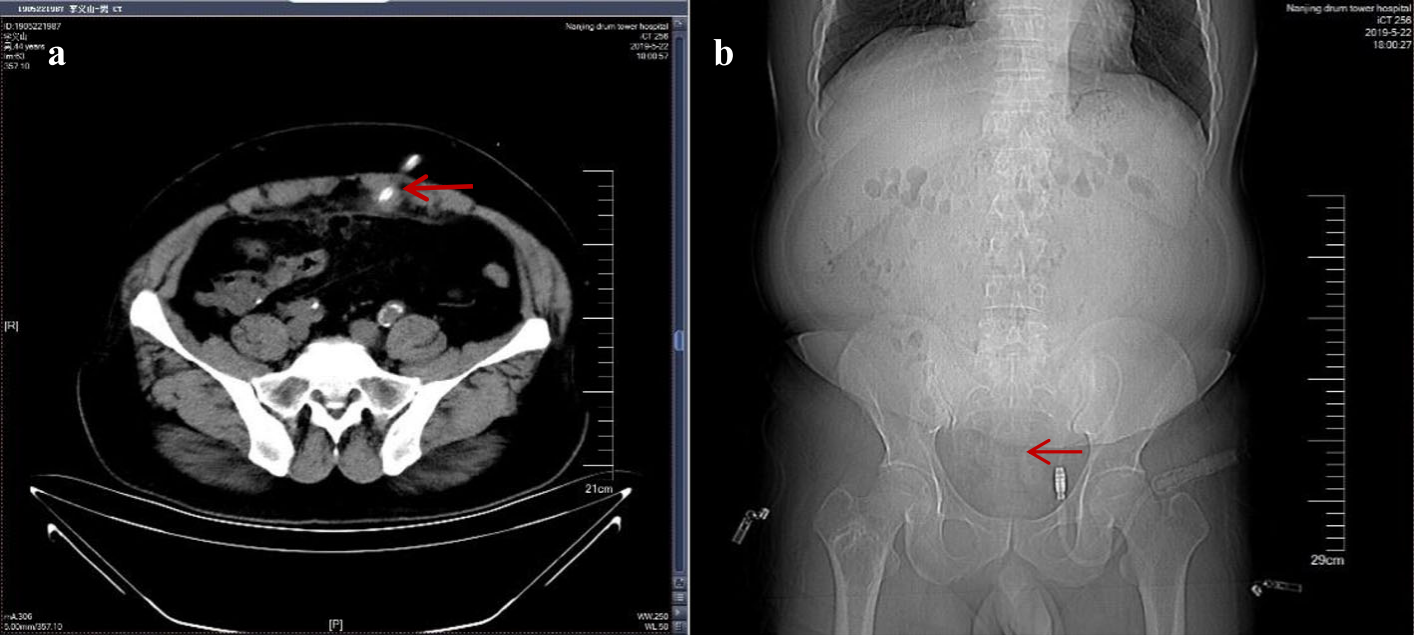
a, b Abdominal computed tomography and radiography showing proper catheter alignment
